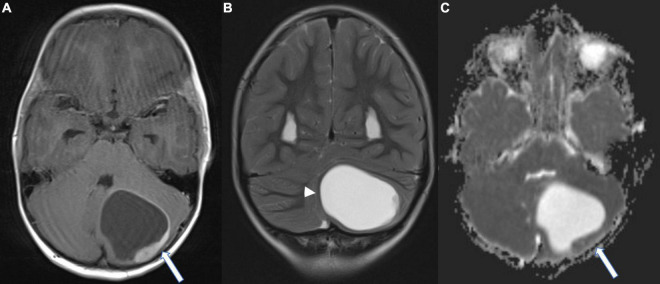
Magnet resonance (MR) images at the time of diagnosis; (A) Axial T1-weighted contrast-enhanced image showing a left cerebellar cystic mass with a peripheral contrast enhancing component (arrow); (B) Coronal T2-weighted image. Note the infratentorial midline shift due to the mass effect of the cystic component (arrowhead). (C) Axial diffusion-weighted image (ADC-Map) demonstrating low ADC values of the solid component (arrow), suggestive of high cellular density.
